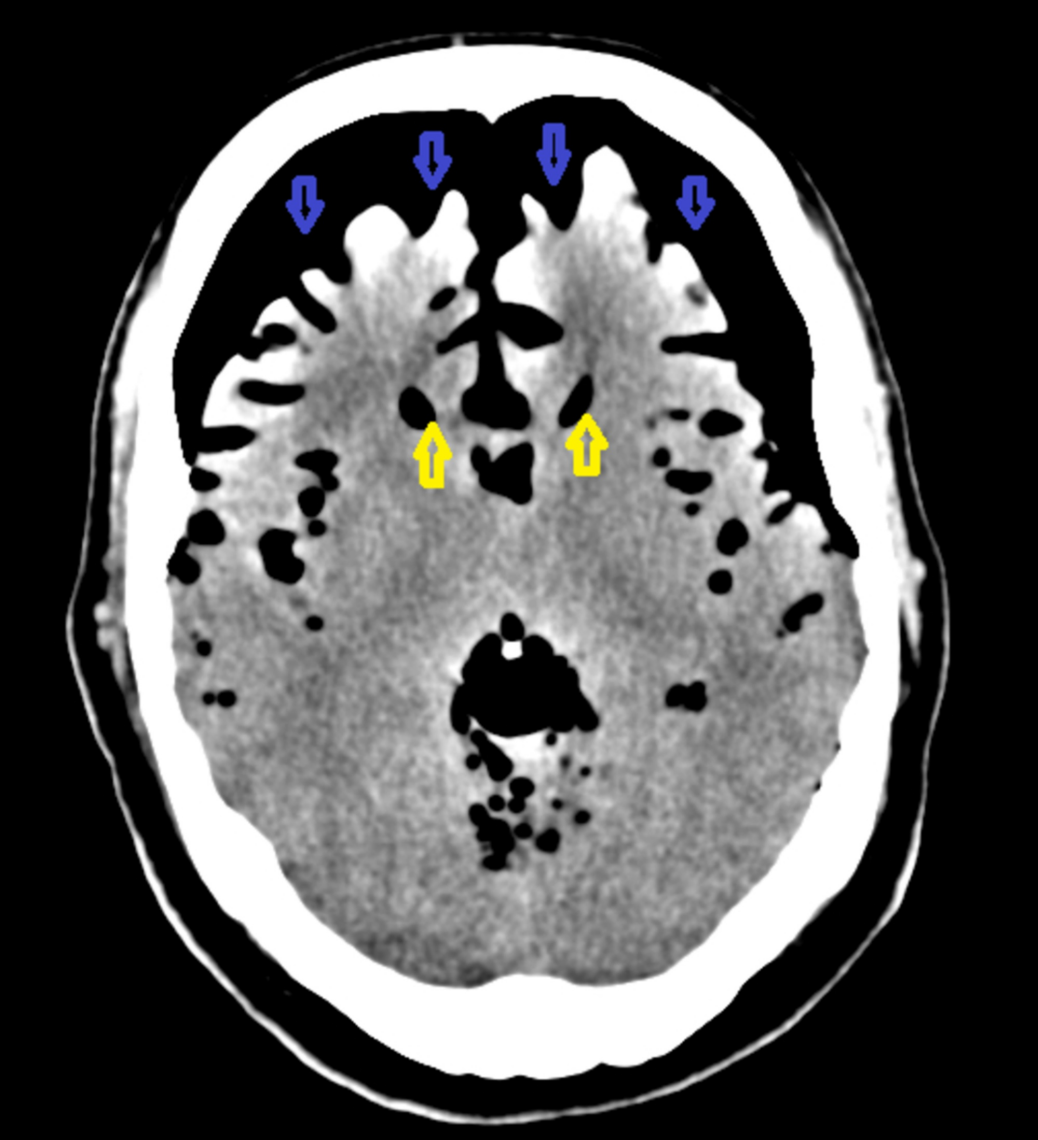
Computed tomography of the head performed on postoperative Day 2 shows the Mount Fuji sign secondary to tension pneumocephalus. The blue-colored arrowhead is pointing towards intracranial air. The yellow-colored arrowhead is pointing towards the slit-like ventricles.
